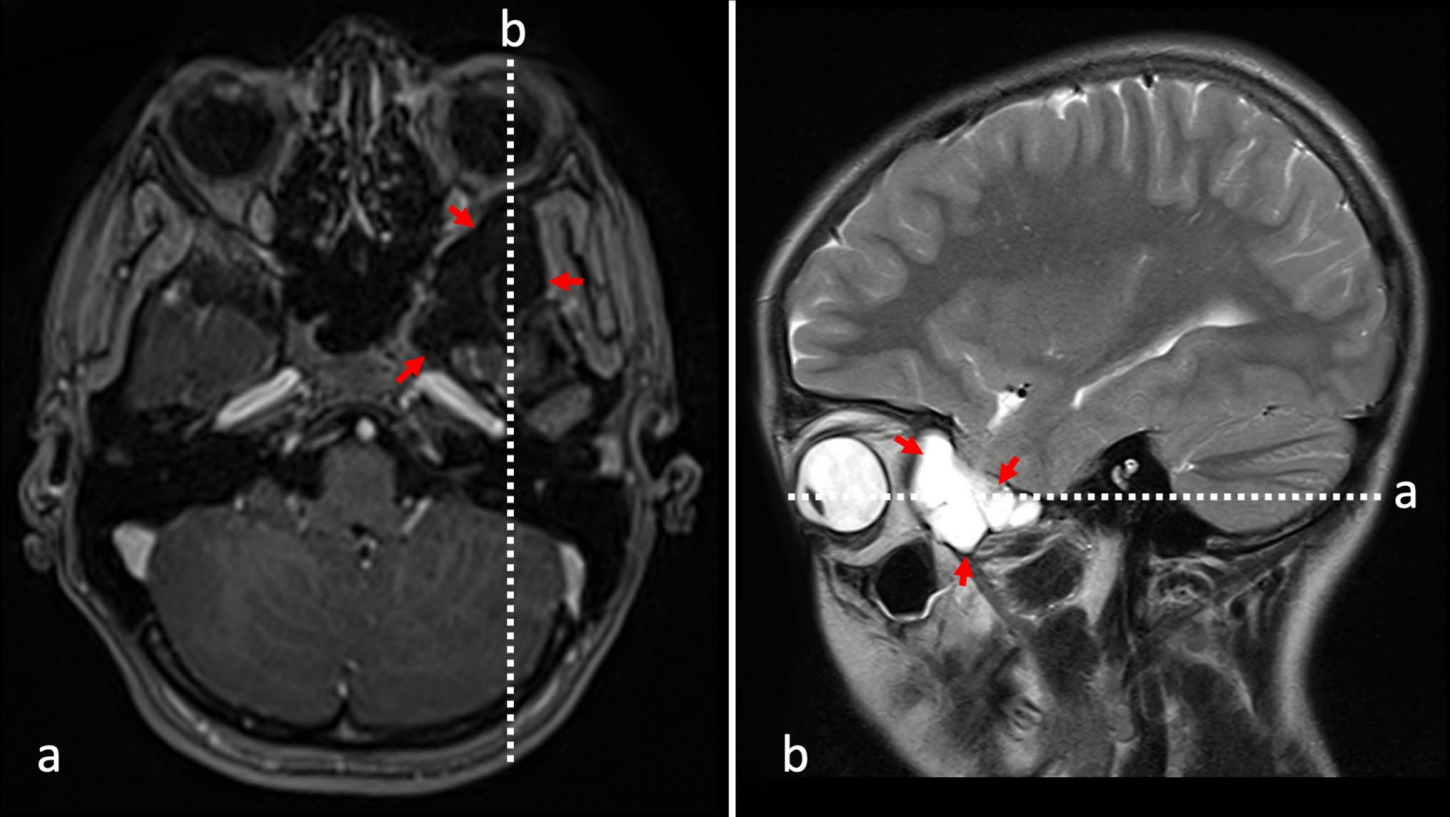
Preoperative head MRI T1W contrast-enhanced scan on axial cross-section (a) with white dotted line marking level of the sagittal cross-section (b) of the T2W image. Cyst is marked with red arrows
